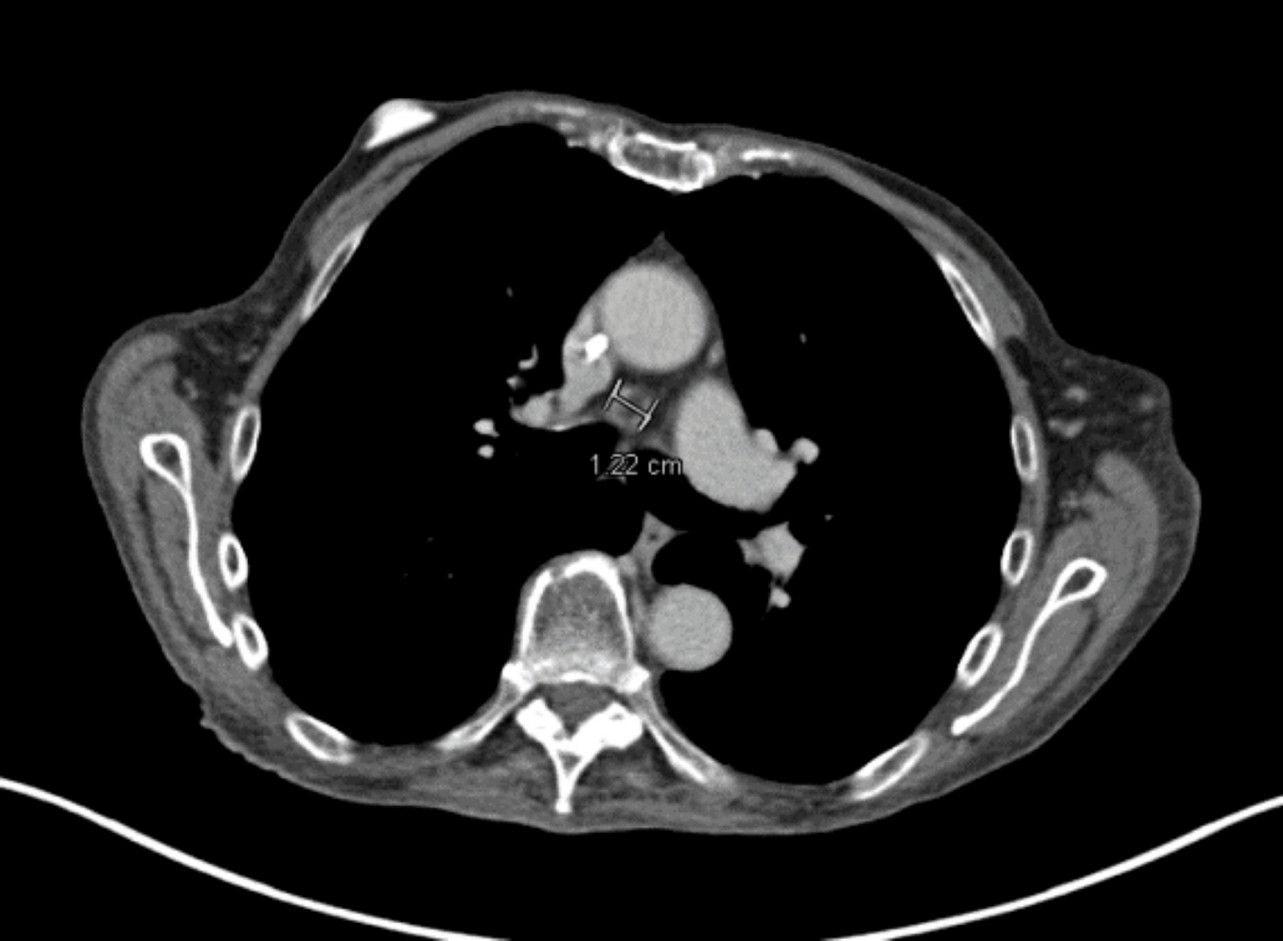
PET/CT scan at eight months after diagnosis Representative image from a repeat PET/CT at eight months, demonstrating a mediastinal lymph node, which had grown from previous imaging to 1.22 cm. Lymphadenopathy had also progressed in the epicardium, retroperitoneal area, and right supraclavicular region (not shown). CT, computed tomography; PET, positron emission tomography
